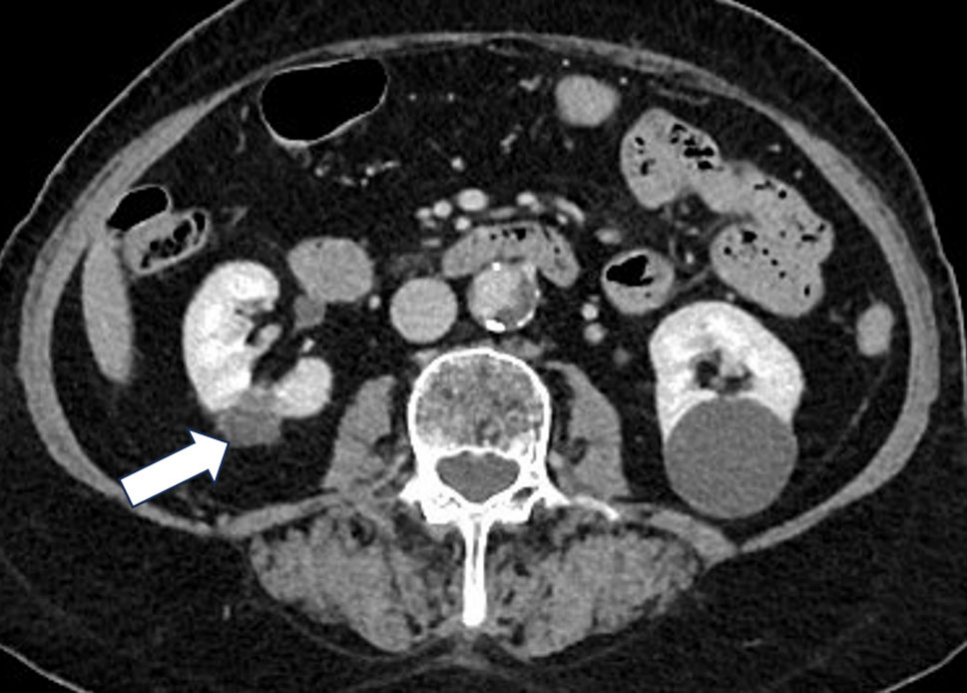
Axial contrast-enhanced CT at 1-year post-histotripsy treatment showed no evidence of residual or recurrent disease within the zone of ablation and rapidly involuting measuring 2 cm
